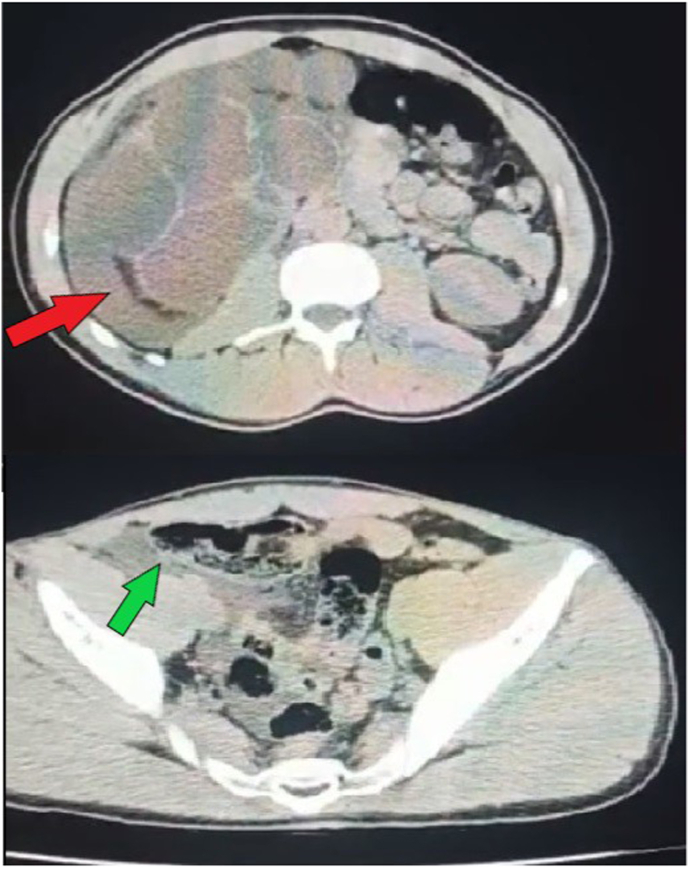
Axial section of a CT abdomen showing perinephric collection (red arrow) and intraperitoneal collection (green arrow). (For interpretation of the references to colour in this figure legend, the reader is referred to the Web version of this article.)
